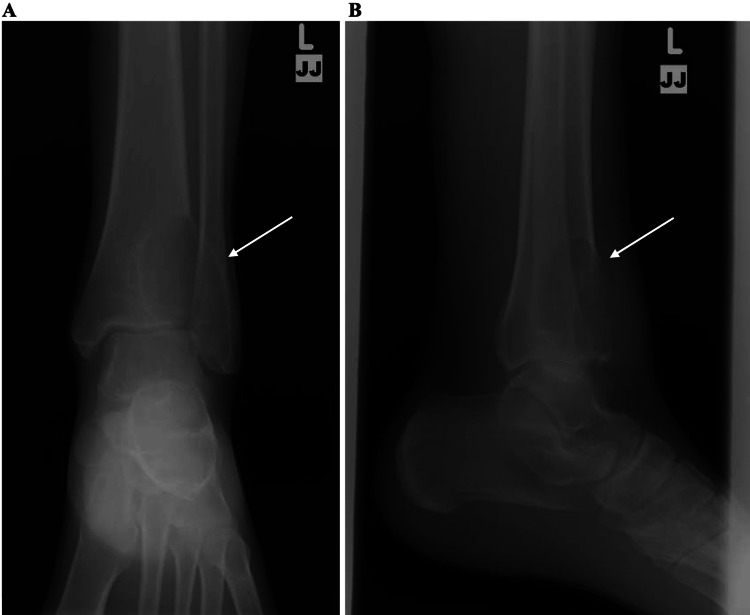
Initial x-rays AP (A) and lateral (B) x-ray views of the left ankle of the patient when she initially presented to the clinic. The lesion is marked with arrows.
